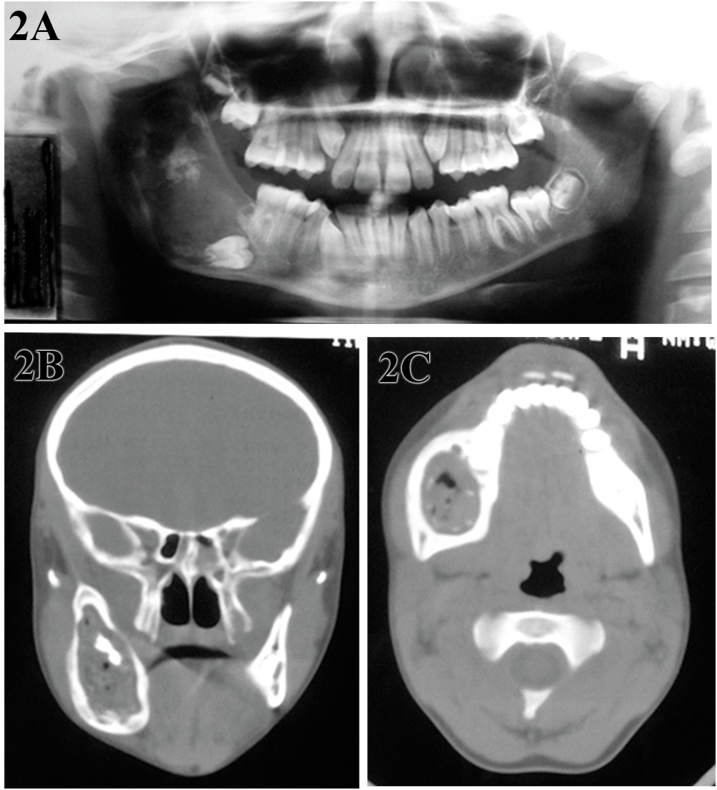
Panoramic radiograph showing a well-defined multilocular radiolucent area in the region from the right lower first molar to the coronoid process, areas of calcification and tooth germ 47 (A). Sagittal (B) and axial (C) computed tomography (CT) cuts showed a well-defined hypodense area, as well as basal cortical bulging of the mandible.
